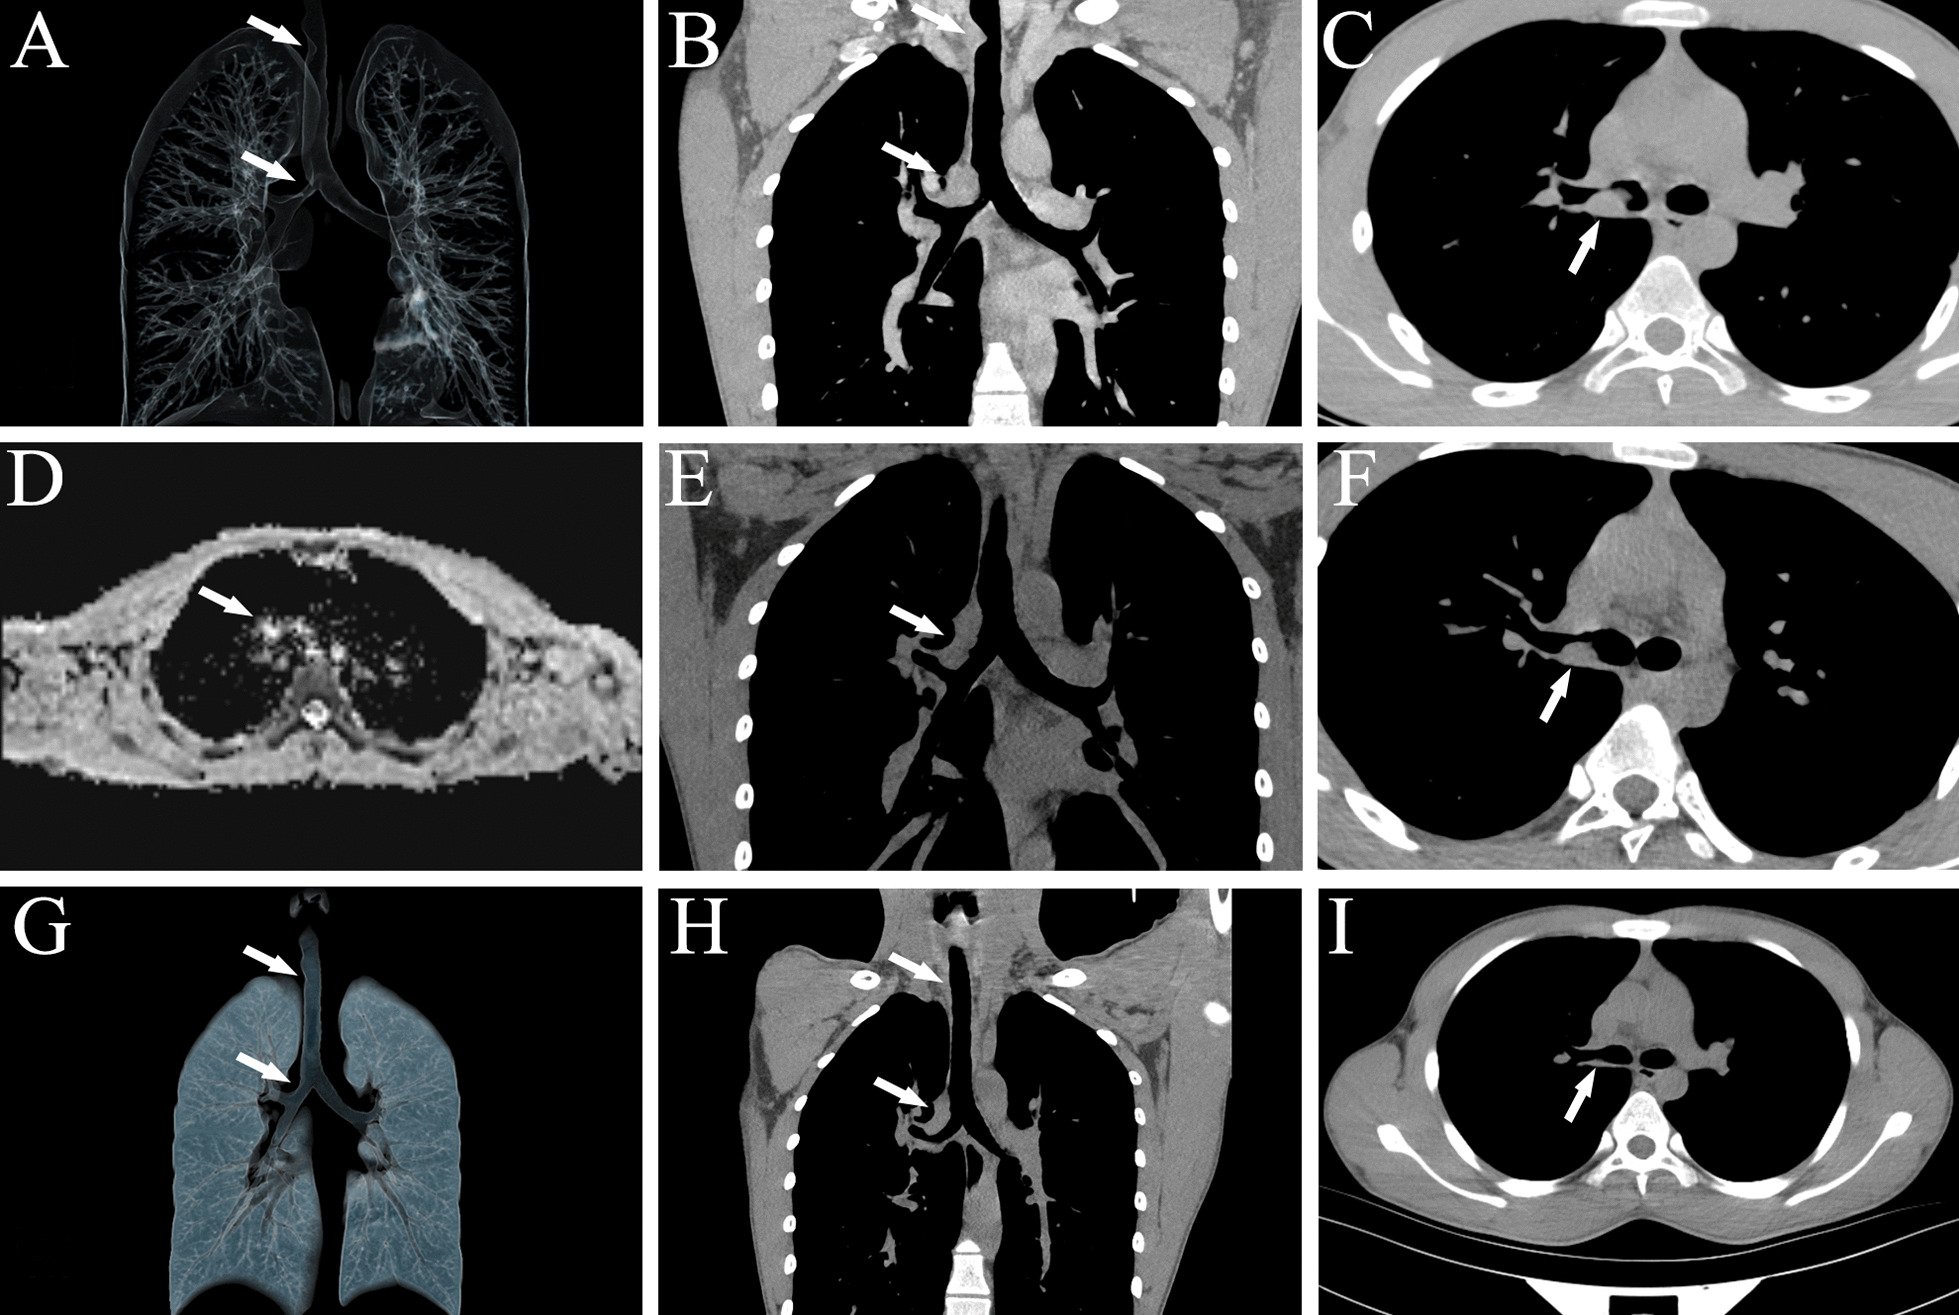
Chest CT image of the RDD patient. A Chest CT three-dimensional image showed 0.9 × 1.0 × 1.5 cm irregular neoplasm (arrows) adhered to the right wall of the trachea and 1.0 × 1.4 × 2.5 cm irregular neoplasm (arrows) adhered to the opening of the right main bronchus resulting in airway stenosis. B Coronal position. C Mediastinal window. D DWI of enhanced chest MRI showed that signals from the neoplasms (arrow) in the trachea and the right main bronchus had been enhanced, and the apparent diffusion coefficient value was about 1.7. E–F The stenosis (arrows) of the trachea and the right main bronchus was significantly improved after two transbronchial resections. G–I After 5 months of follow-up, the trachea and the right main bronchus were unobstructed (arrows)
